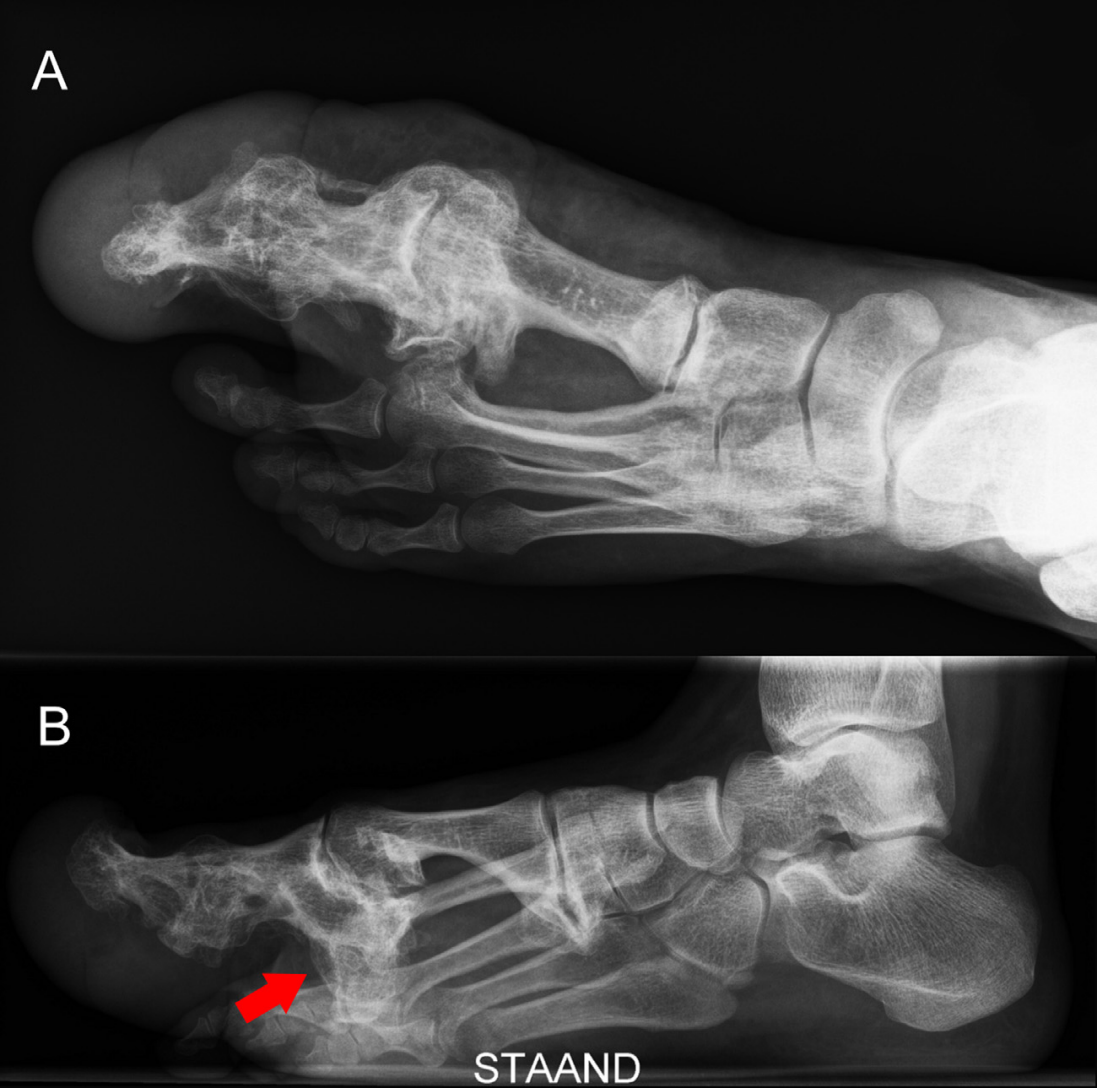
Conventional radiographs of the left foot of case 2. Status after amputation of the second toe through the MTP joint and soft tissue debulking of the first toe in 1993. A: A-P radiograph showing significant bone overgrowth and deformation of the phalanxes and around the MTP joint of the first toe. B: Lateral radiograph in standing positions, notice the substantial bony formation on the plantar side of the foot (arrow) and thereby tilt of the foot.
